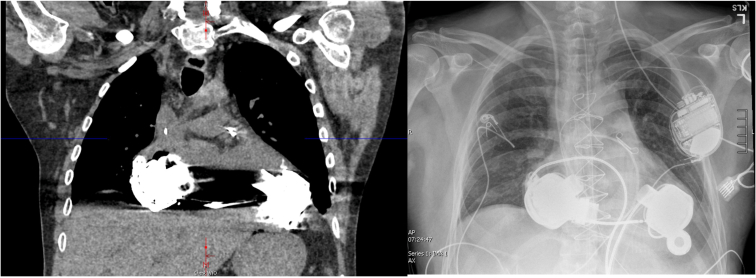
Postimplant computed tomography scan (*left*) and radiograph of the chest (*right*).7.The LVAD outflow graft is trimmed to an appropriate length. A partial occlusion clamp is placed on the ascending aorta, and a rounded aortotomy is created. A circumferential anastomosis is performed with a running 3-0 PROLENE RB 1. The aorta is deaired and the LVAD also is initiated at 4000 rpm. We wean the patient off cardiopulmonary bypass and bring them up on biventricular flow with the goal of achieving full flow in both VADs. We err on the side of greater flow in the LVAD to allow for slightly more volume in the right heart to Prevent suction events. We balance the speeds on both VADs using the ventricular septum on transesophageal echocardiography to avoid bowing.8.After sternal closure, 3 separate no. 5 ETHIBOND sutures (Ethicon) are used to suspend the RVAD chassis in an optimal position relative to the chest wall with distinct fixation points at the sternum around the ribs. This prevents suction to the atrial septum, which is a common problem if this step is omitted.
